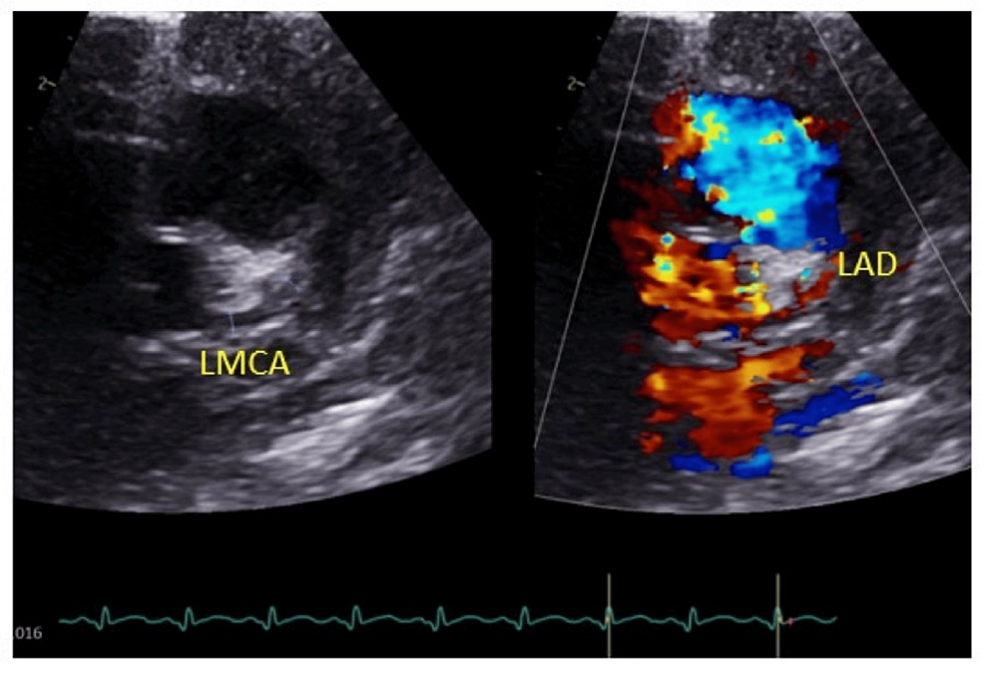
An echocardiogram (ECHO) showing prominent LMCA LMCA: left main coronary artery, LAD: left anterior descending artery
